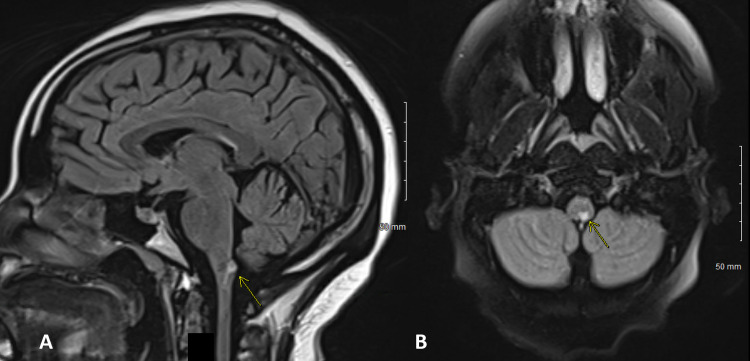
MRI FLAIR images in the sagittal (A) and axial (B) planes demonstrating the lesion at the cervical-medullary junction (arrows) MRI: magnetic resonance imaging; FLAIR: fluid-attenuated inversion recovery
